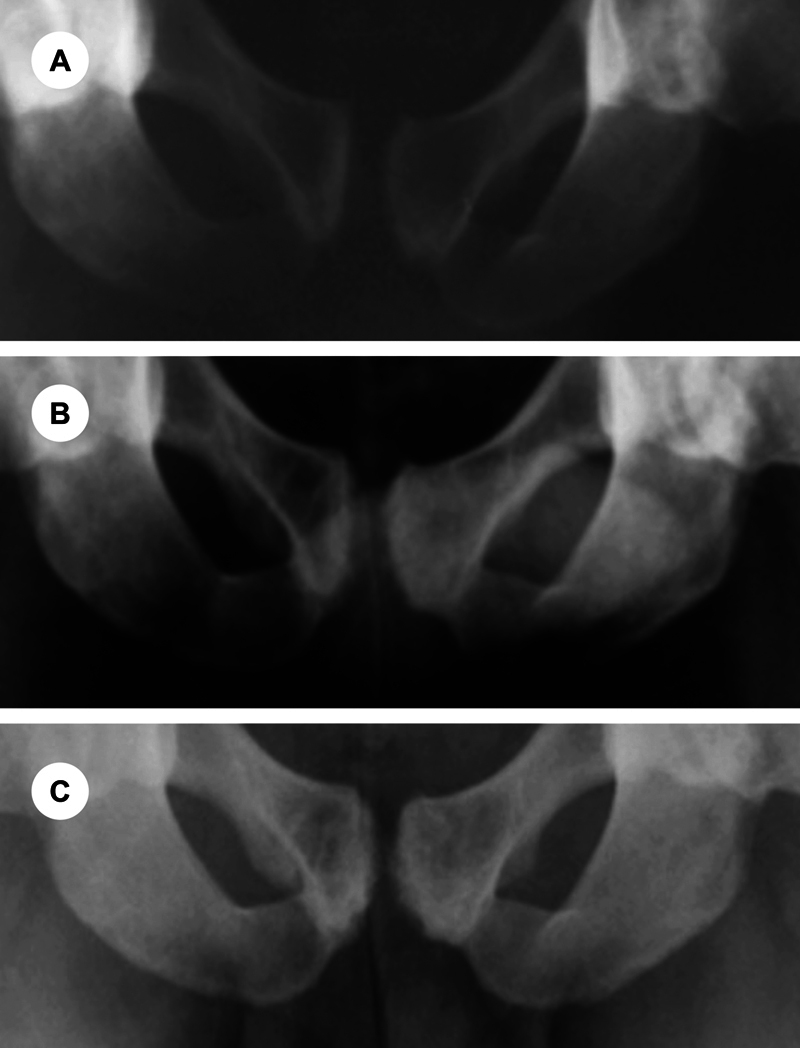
Serial radiographs of the pubic symphysis: (
